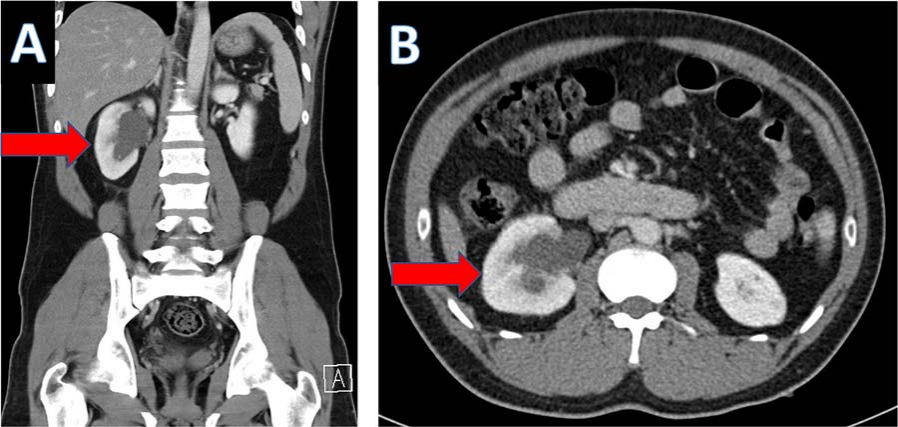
CT scan showing hydronephrosis of the right kidney (A: coronal; B: axial).
